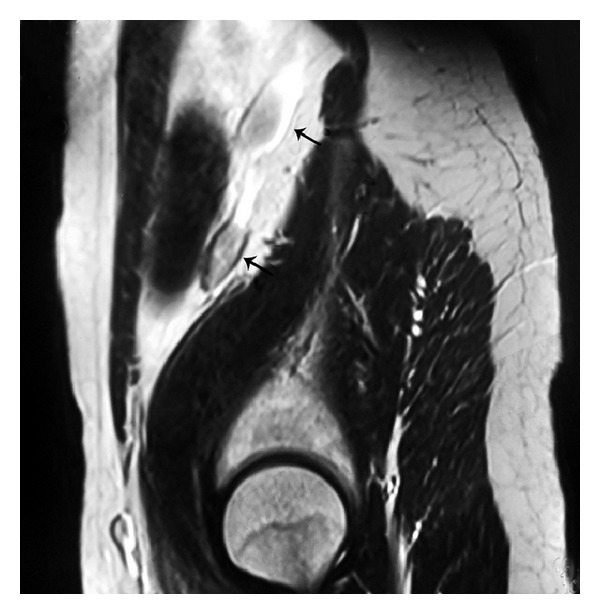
MRI T2, sagittal cuts showing 2 oblong masses in the left retroperitoneum.
